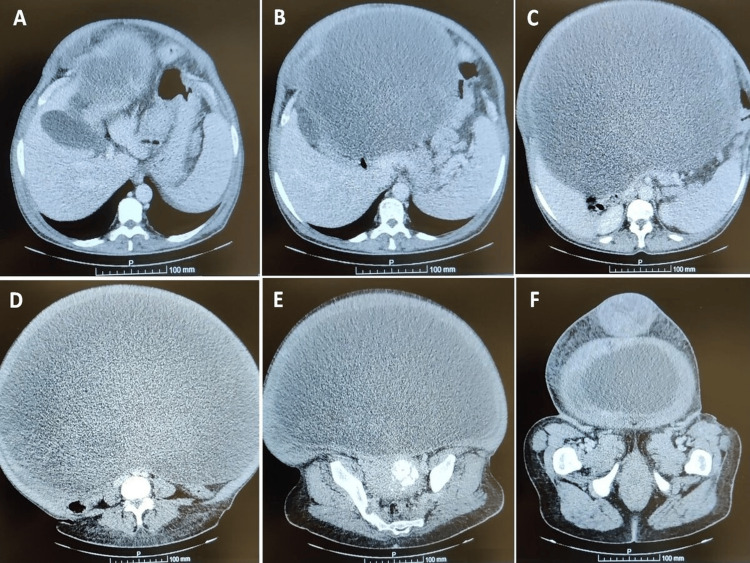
Multiple sections of the thoracic-abdominopelvic CT scan, oriented in a cephalocaudal direction.
